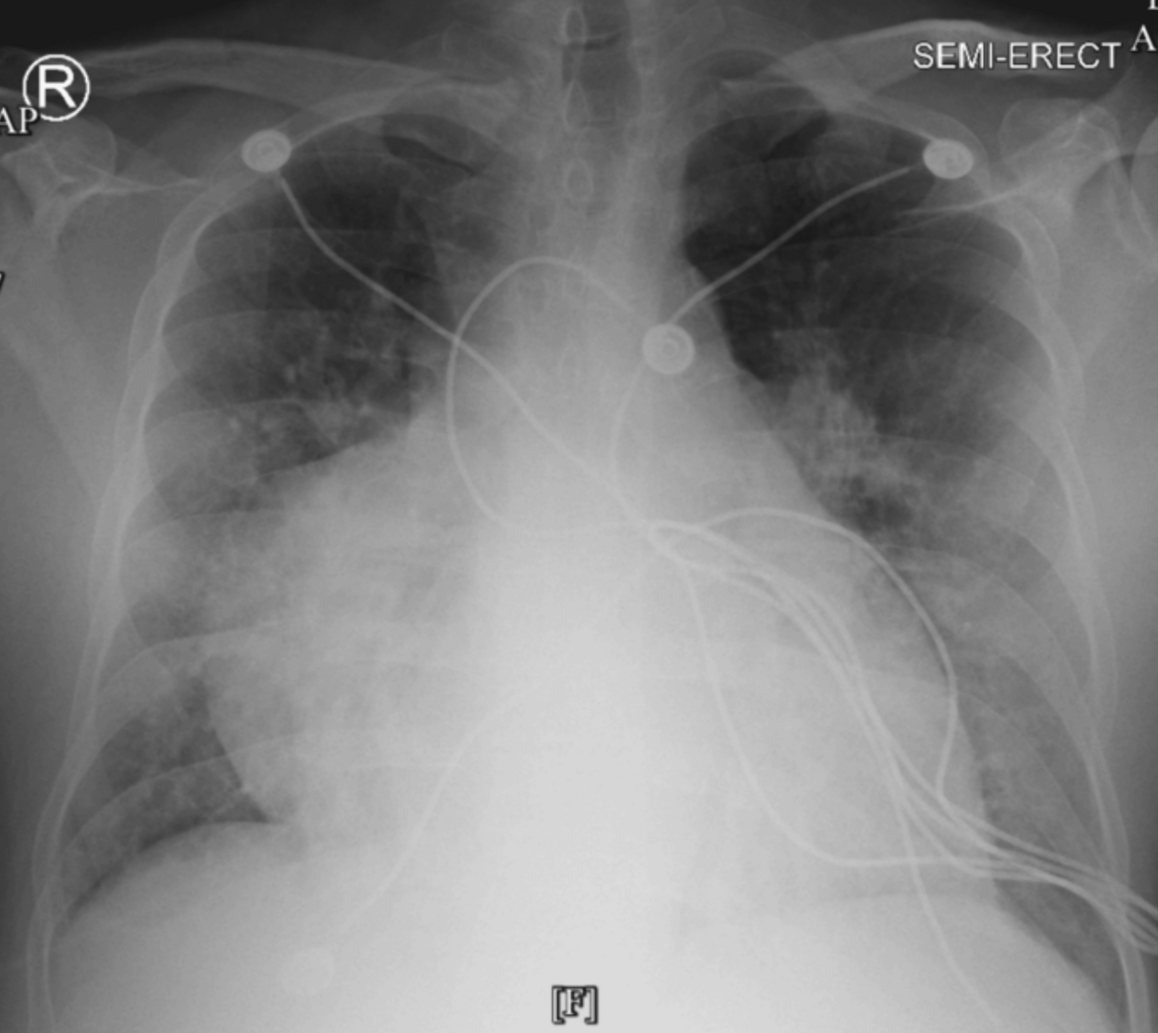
Admission chest radiograph demonstrating prominent vascularity bilaterally
